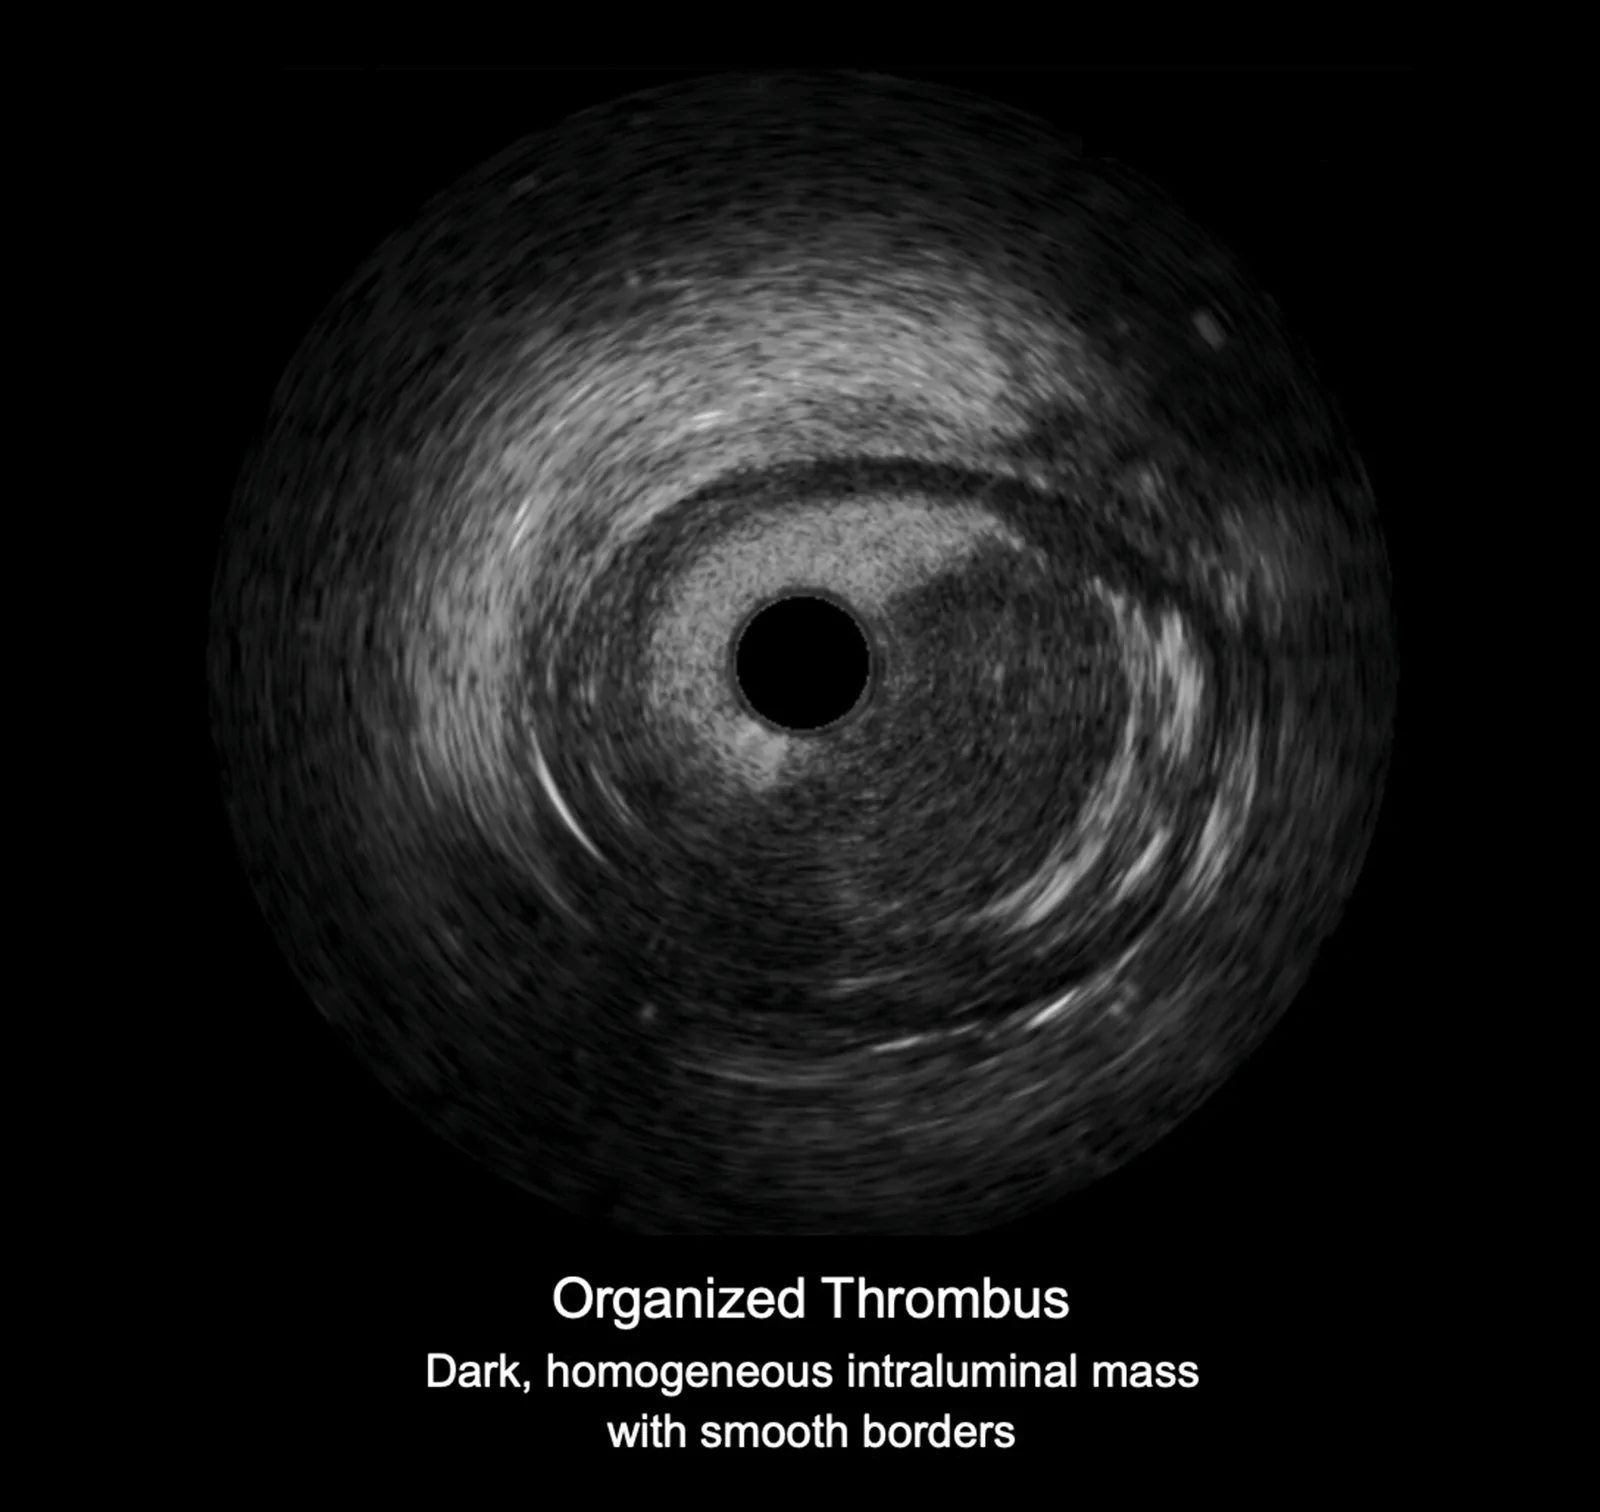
Intravascular Ultrasound Demonstrating Thrombus Composition in Case 1 Intravascular ultrasound imaging of the proximal left circumflex artery following wire crossing and balloon angioplasty. (Left) Acute thrombus appears as a bright intraluminal mass with well-delineated borders and no acoustic shadowing (red arrows), consistent with a fresh, fibrin-poor platelet-rich thrombus. (Right) Early organizing thrombus (asterisk) appears as a gray intraluminal mass with a speckled echotexture and irregular borders (outlined in white), reflecting early fibrin crosslinking.
